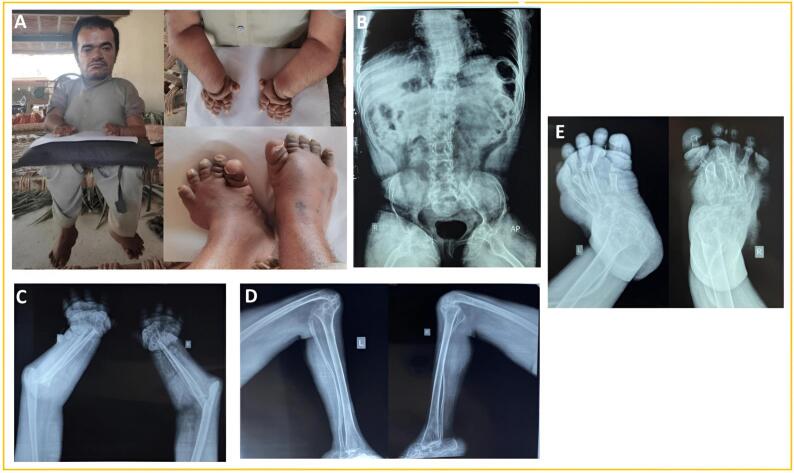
(A) Images of the affected individual IV-2 displaying the same features of MONA syndrome as his elder brother (B–E) Radiographs of the patient (IV-2) at the age of 26 years. (B) Mild scoliosis of the spine, irregularity of the femoral heads, loss of left ischiopubic ramus. (C) Severe destruction of proximal and distal ends of ulna and radius, deformed metacarpals and missing carpal and phalangeal bones. (D) Severely distorted fibula, destruction of the ankle joint. (E) Loss of talar bones, deformed metatarsal bones with distal tapering in some metatarsals, deformed and partly missing phalangeal bones.
